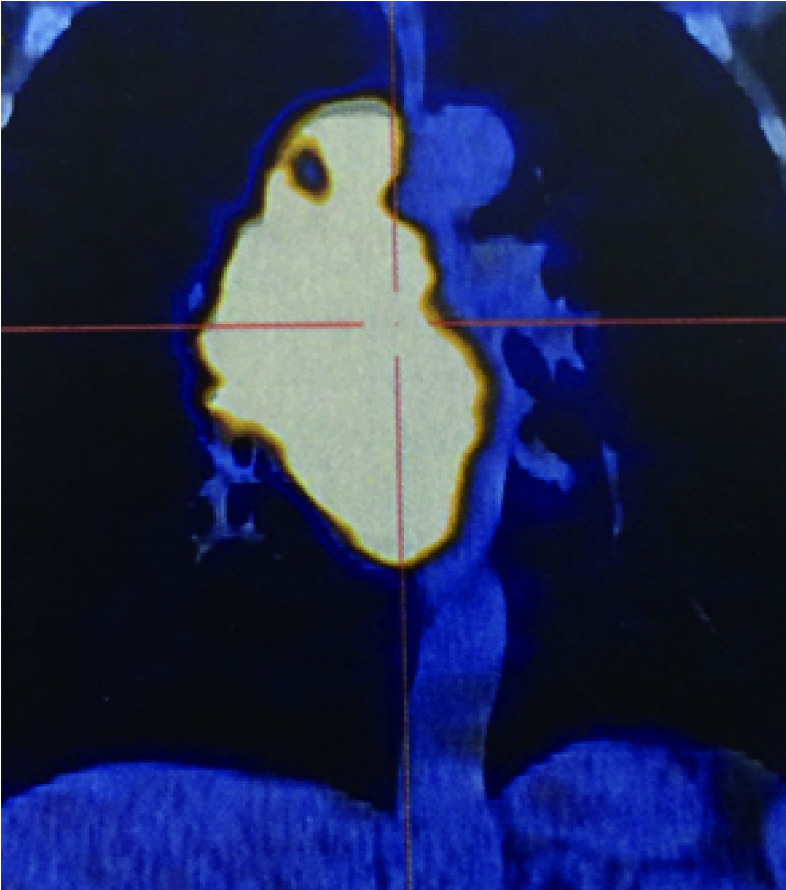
恶性胸腺瘤侵犯上腔静脉的PET/CT征象 PET/CT images of malignancy-related superior vena cava syndrome.PET/CT: positron emission tomography/computed tomography.
